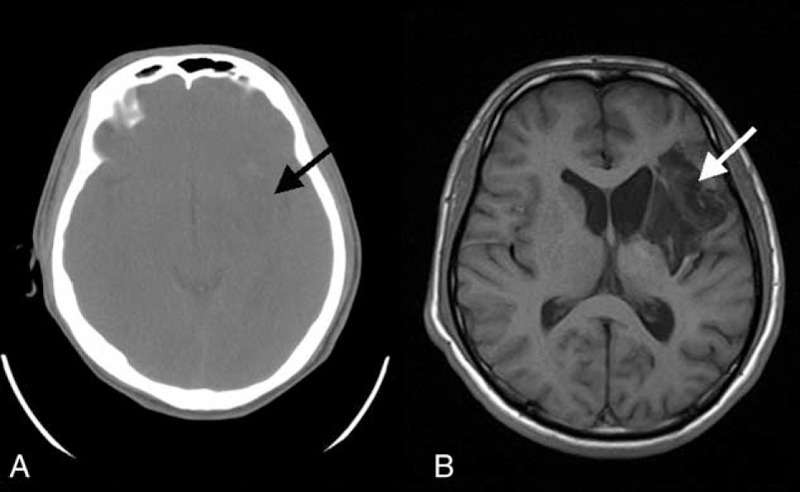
Brain CT (A) and MRI (B) showing a large infarct area (arrow) in the left basal ganglia, corona radiate, and temporal lobe. CT = computed tomography, MRI = magnetic resonance imaging.
